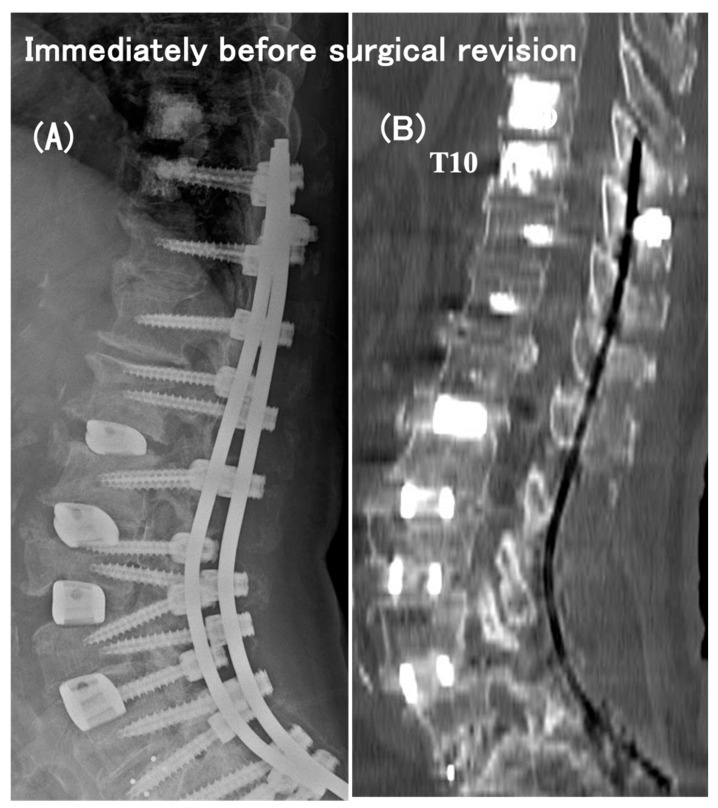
The plain radiograph (A) and a sagittal reformatted CT scan (B) obtained immediately before surgical revision for paraparesis of modified Frankel grade C, which developed as early as 2 months after the initial operation. These images indicated neither a PJK increase nor a vertebral fracture nor an implant failure.
